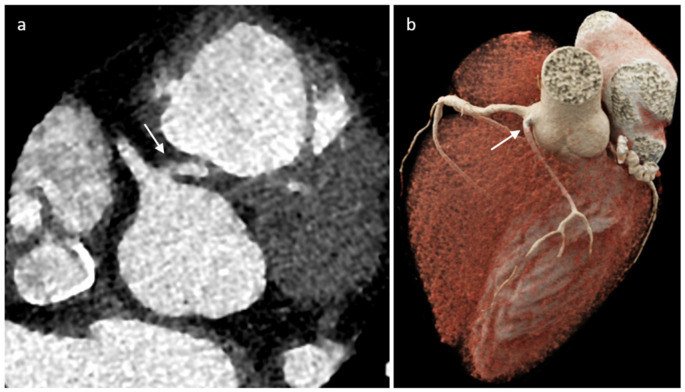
Single coronary artery from right coronary sinus with a malignant course of the left coronary artery (arrow) at axial (a) and volume-rendering (b) computed tomography images.
